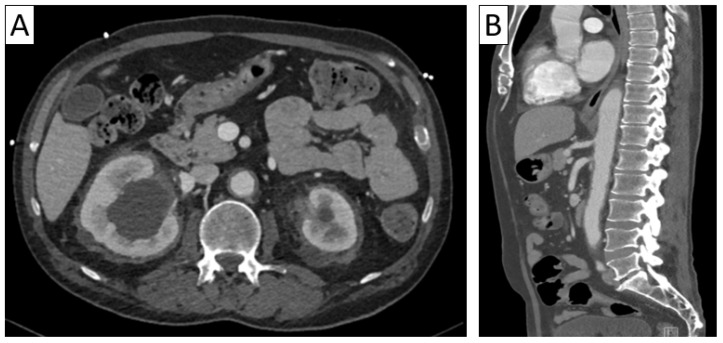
Differential diagnosis of vasculitis—Erdheim–Chester syndrome. Axial abdominal CT (A) with sagittal reformatting (B) showing diffuse retroperitoneal periaortic and perirenal tissue infiltration (“coated aorta” sign and “hairy kidneys”) in a patient with multiorgan affection (cerebral, osseous, renal, and pulmonary).
